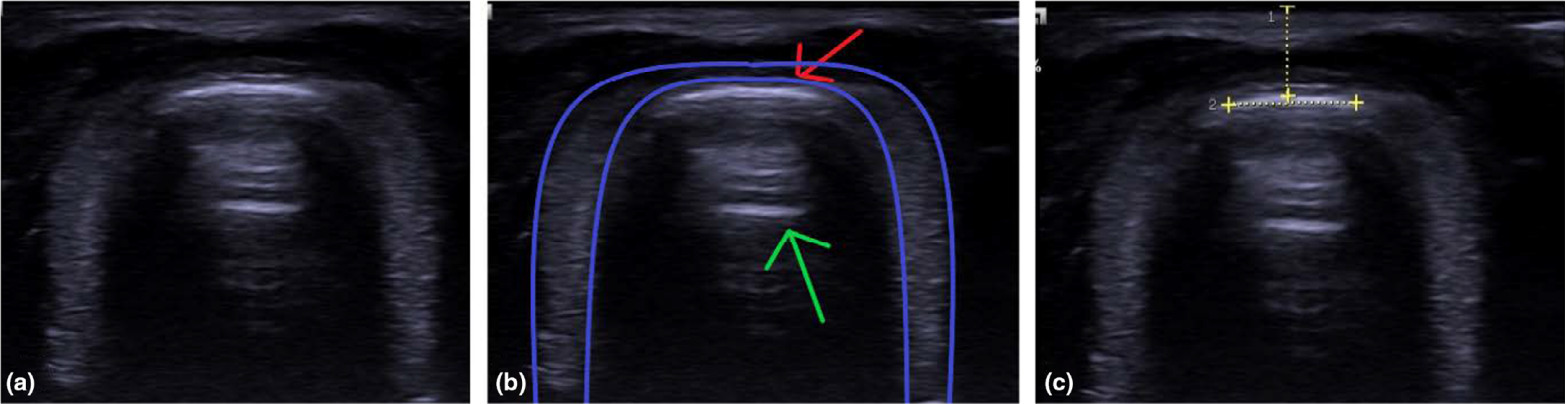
(a) Ultrasound image showing the transverse view of the CTM. (b) The CTM is seen as a white horizontal line formed at the mucosa–air interface (red arrow). White lines deep to the CTM are reverberation artefacts (green arrow). (c) Depth and width of the CTM are measured as shown.
